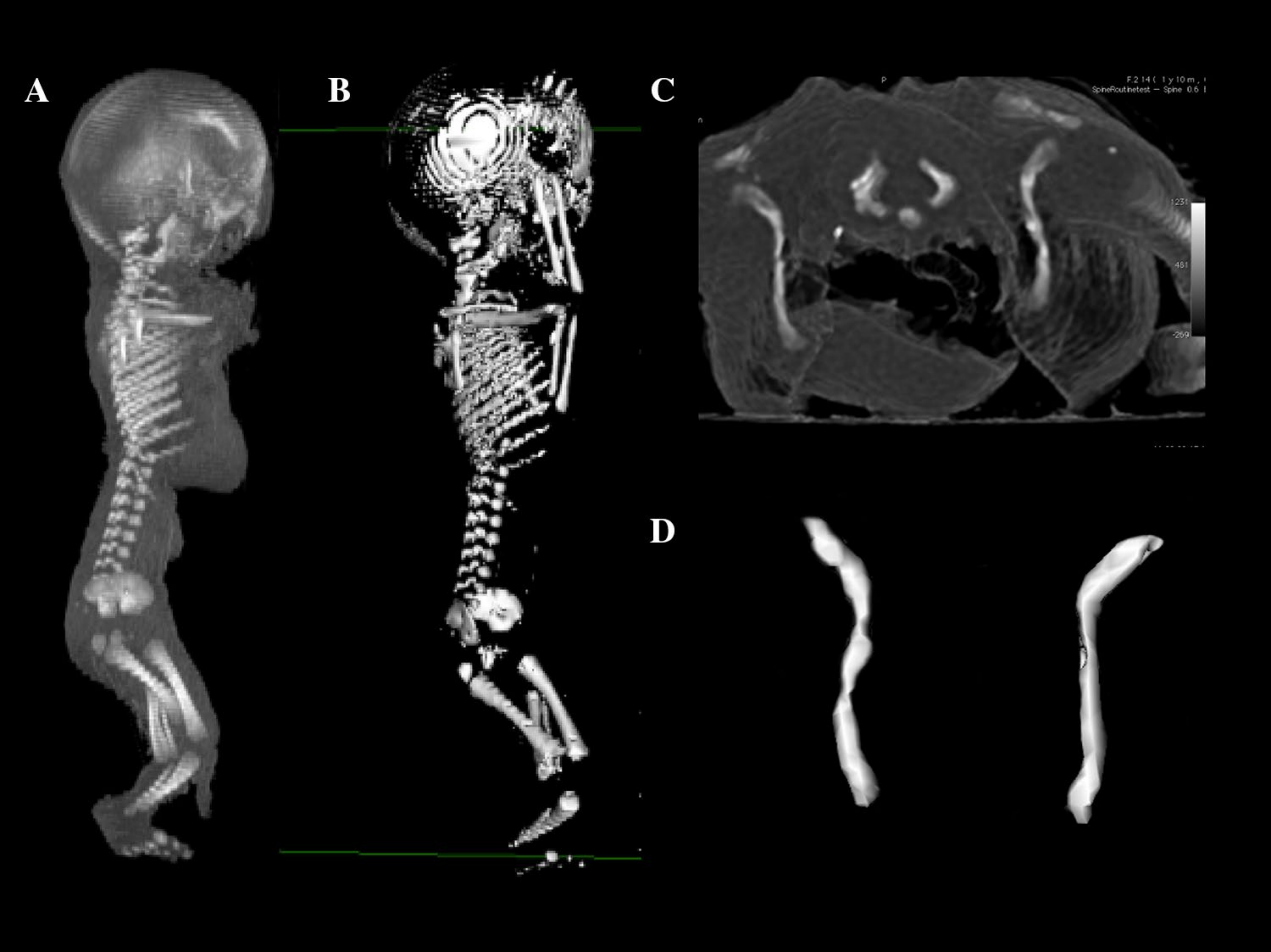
CT of a male fetus aged 24 weeks in the sagittal projection recorded in DICOM formats (a), 3D fetus reconstruction in the sagittal projection (b), its clavicle in the horizontal projection (c), and 3D clavicle reconstruction in the horizontal projection assessed by Osirix 3.9 (d)
